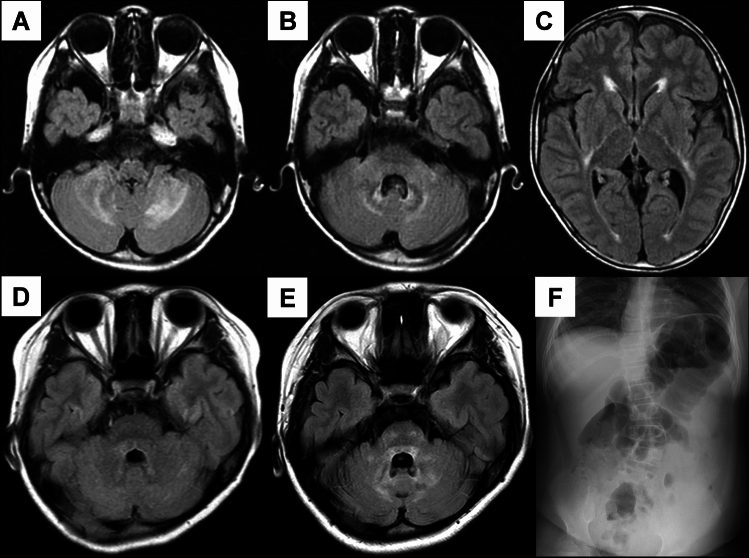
Brain MRI and spinal imaging of Patients 4 and 5 with classical GAN. A–C Axial FLAIR images of Patient 4 at 8 years old revealing high-intensity lesions in the periventricular white matter and bilateral cerebellar hemispheres, along with brainstem atrophy. D–E Axial FLAIR images of Patient 5 at 9 (D) and 14 years old (E). At 9, only a mild hyperintense lesion was observed in the periaqueductal gray matter of the midbrain. By 14 years of age, hyperintense lesions had expanded to the bilateral cerebellar hemispheres. F Abdominal radiograph of Patient 5 at 9 years old revealing scoliosis and bowel gas accumulation. FLAIR, fluid-attenuated inversion recovery
